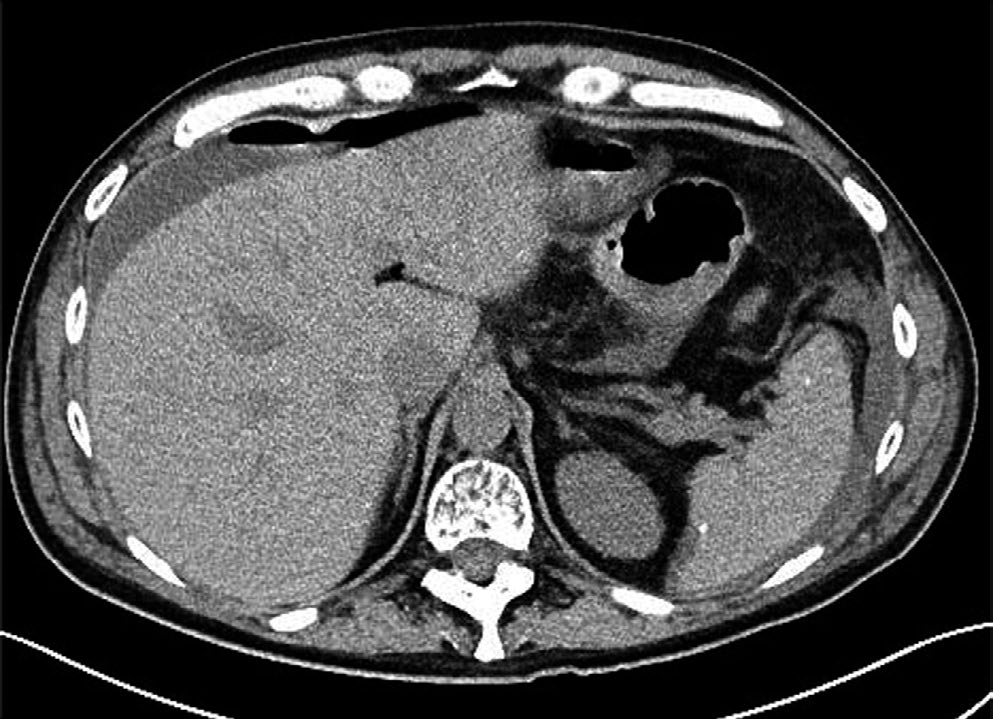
Bowel perforation in a CT scan in one of the patients Courtesy of Benjamin M. Howe
